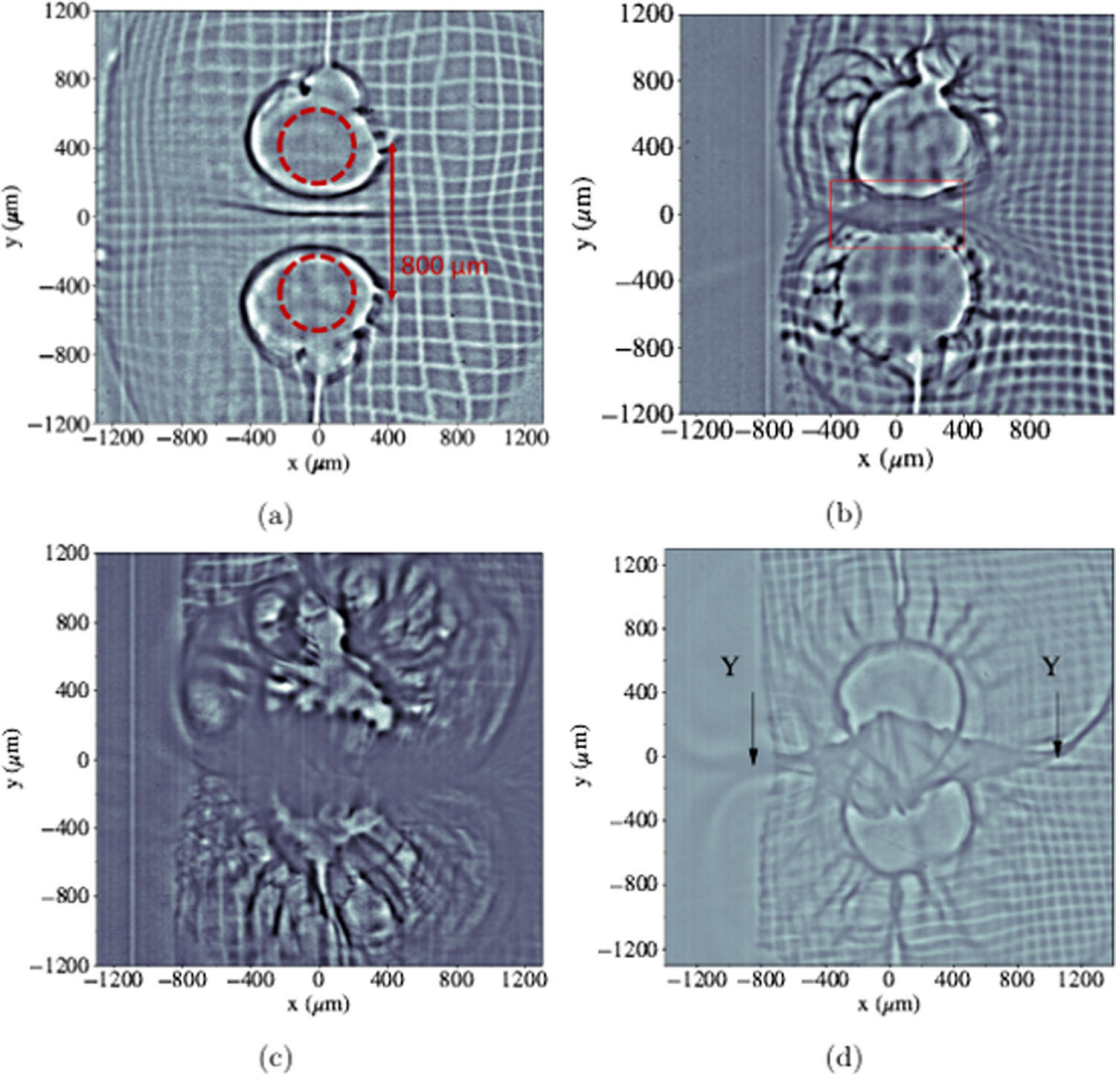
Experimental proton radiographs at different probing
